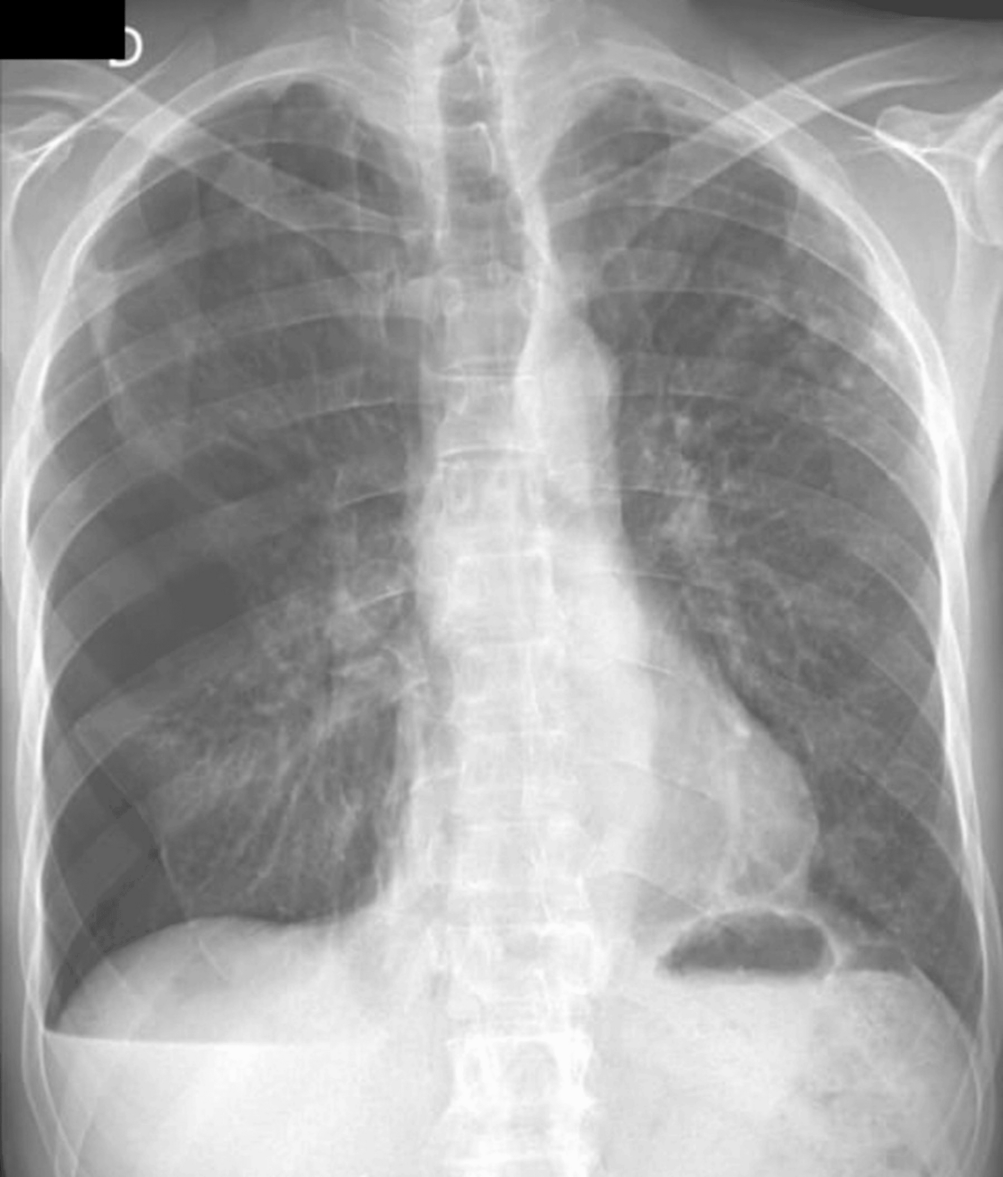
Thoracic X-ray in the postero-anterior (PA) view demonstrating basal pneumothorax
